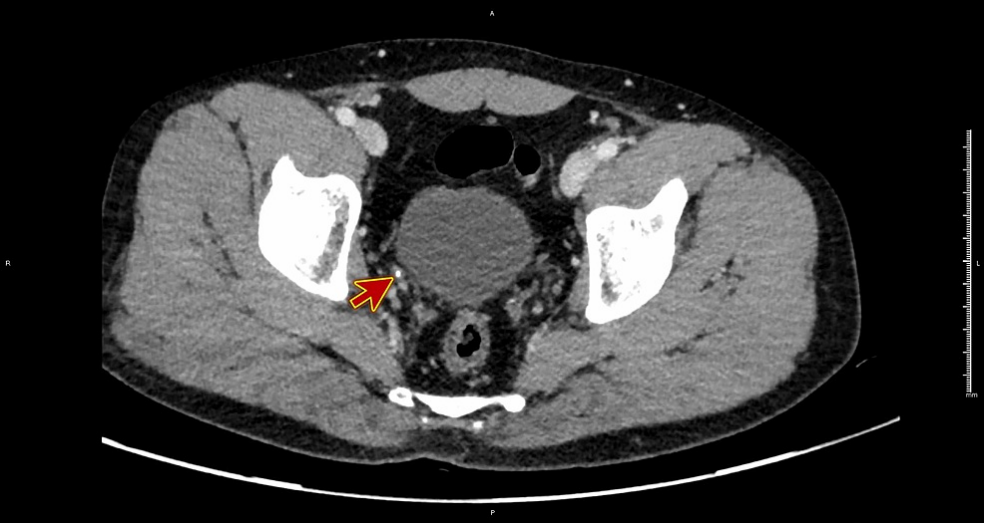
CT scan of the abdomen and pelvis (plain) showing a 3.2 mm calculus in the distal part of the right ureter (red arrow).
